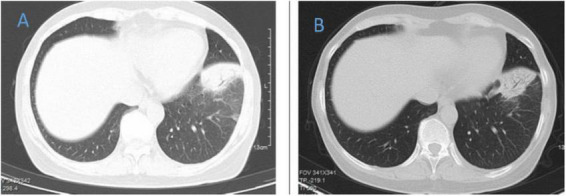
Chest CT of patient 4. (A) Before treatment on 2021.10.26. (B) The follow-up imaging at 1 week post-treatment showed stable findings compared to the pre-treatment scans.
